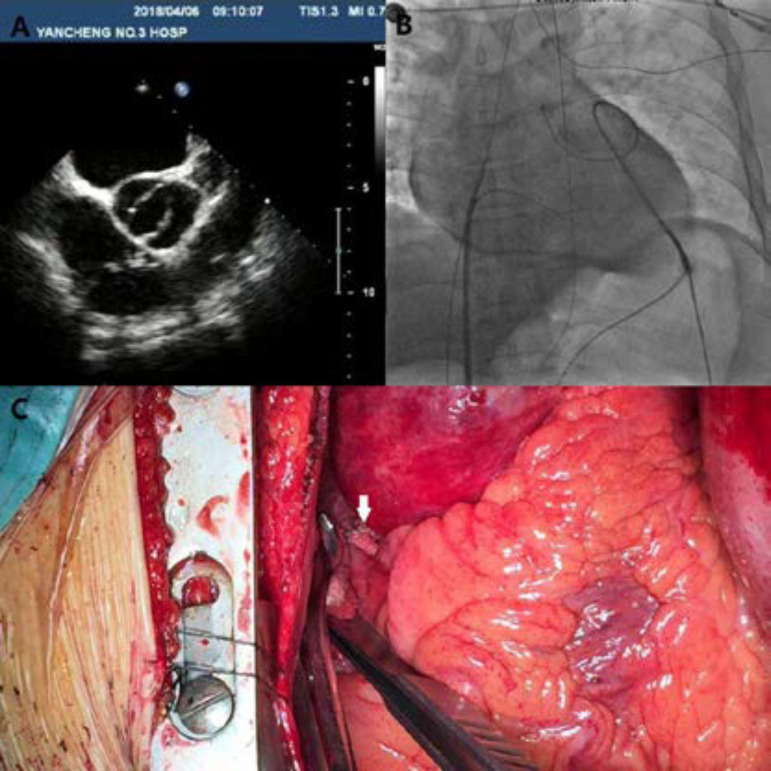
Images of transesophageal echocardiogram, chest X-ray and thoracic surgery. A: Transesophageal echocardiogram reavealed congenital bicuspid aortic valve. B: Guidewire was found to enter the thoracic aorta and pericardial drainage was performed via apical puncture with the Seldinger technique. C: Hematoma in the region of right atrium roof and the thoracic aorta were seen and the ongoing bleeding was managed by surgical repair (white arrow).
